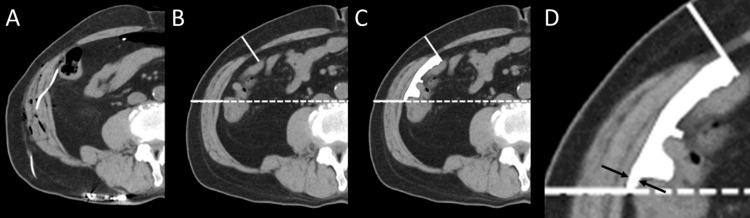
Method for Measuring the Minimum Distance Between the Transversus Abdominis Muscle and Intestine at the Ventral Catheter Insertion Site (A) Postoperative abdominal CT scan showing the ventral catheter insertion site following LP shunt surgery. (B) Preoperative CT scan showing the ventral catheter insertion level. (C) Preoperative CT scan revealing the space (white area in C) between the transversus abdominis muscle and the intestine at the same level. (D) Measurement of the minimum distance between the transversus abdominis muscle and intestine (black arrow). LP: Lumboperitoneal
